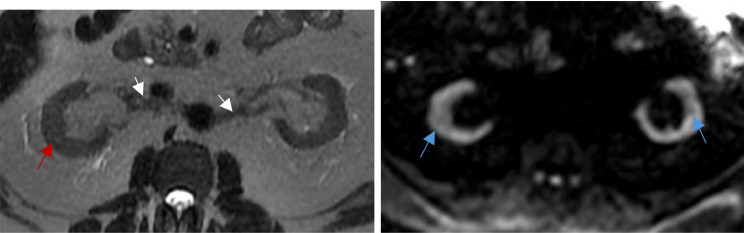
Axial T2 DWI-MRI with mild homogeneous T2 hypointensity (red arrow), no fluid in the renal collective systems (white arrows), and diffuse cortical restricted diffusion (blue arrows)
